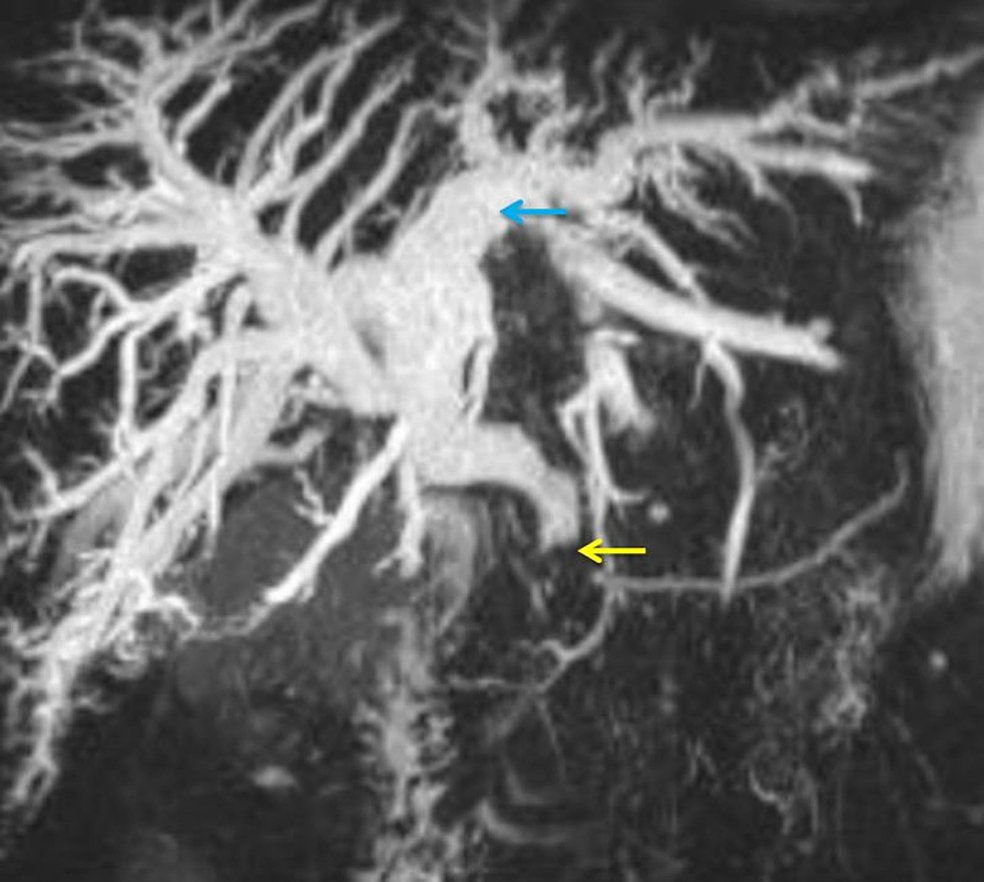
Magnetic resonance imaging (reconstructed T2 image) showing gross dilated intrahepatic biliary radical dilatation (blue arrow) with an abrupt cut-off of the dilated bile duct due to external lymph nodal compression (yellow arrow).
